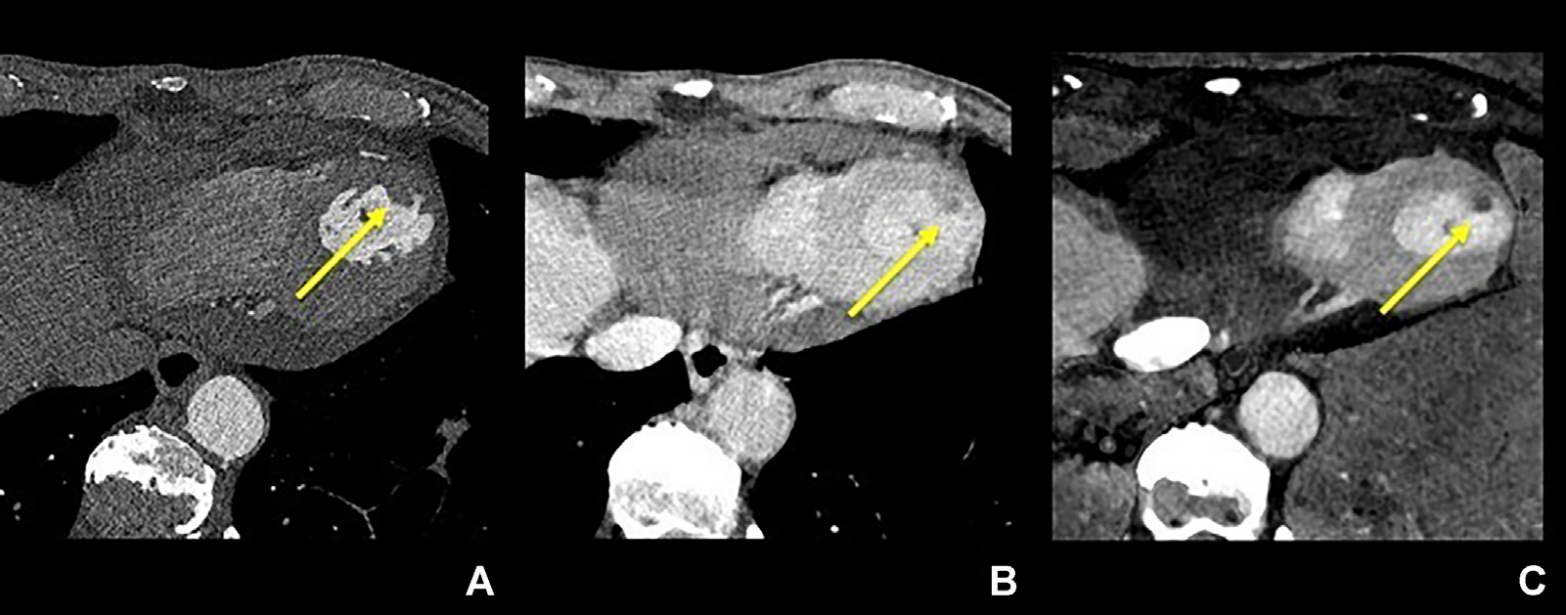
The smaller thrombus adherent to the myocardium on axial images scanned with photon-counting-detector CT: the coronary arterial phase (A), the delayed phase scanned 50 seconds after a contrast media injection (B), and the iodine map made from the delayed phase (C). Note that the iodine map also better visualizes the thrombus than other 2 images because of the higher contrast between the thrombus and myocardium.
